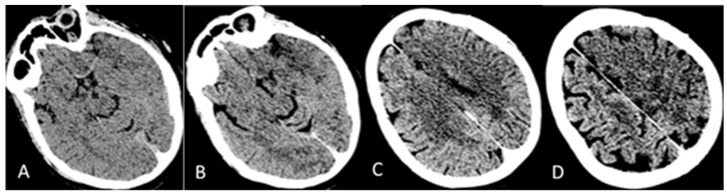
Brain CT on day one. (A) Vessel hyperdensity on the left of thrombotic significance and bifurcation of the siphon, the M1, and the A1–A2 tracts; (B–D): Cortico–subcortical temporo-insular and fronto-cingulum-parietal hypodensity. ASPECT score 4.
